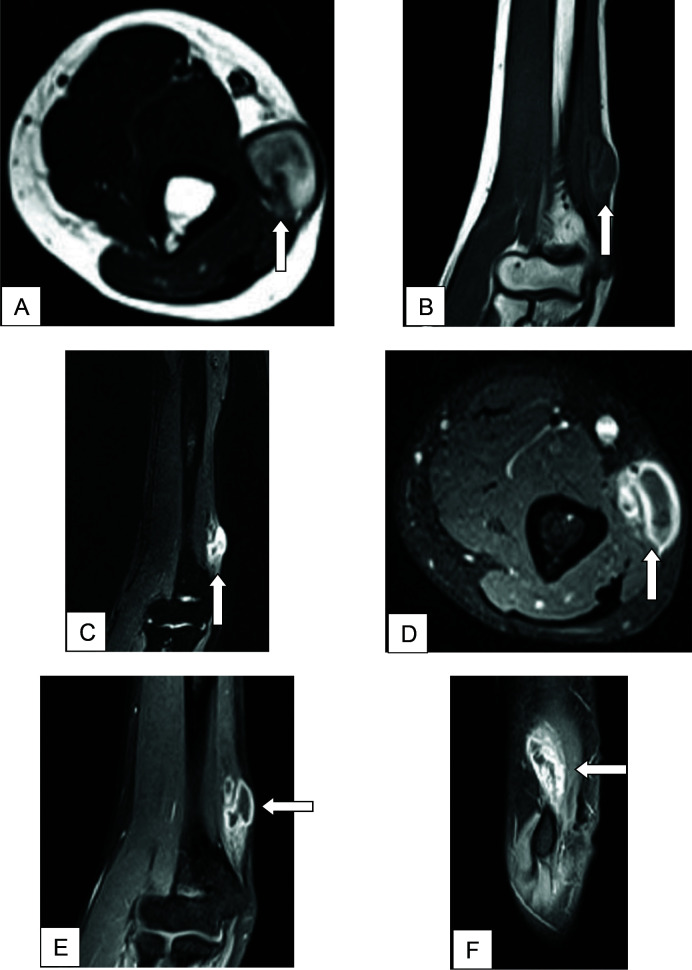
(A) Axial T2-weighted, (B) Coronal T1-weighted, (C) Coronal STIR, (D) Axial fat-suppressed T1-weighted post-contrast, (E) Coronal fat-suppressed T1-weighted post-contrast, and (F) Sagittal fat-suppressed T1-weighted post-contrast MRI images. T1 isointense, T2/STIR hyperintense lesion (thick white arrows) noted in the medial aspect of the distal right arm in the subcutaneous plane showing peripheral rim enhancement on T1 post-contrast scan.
